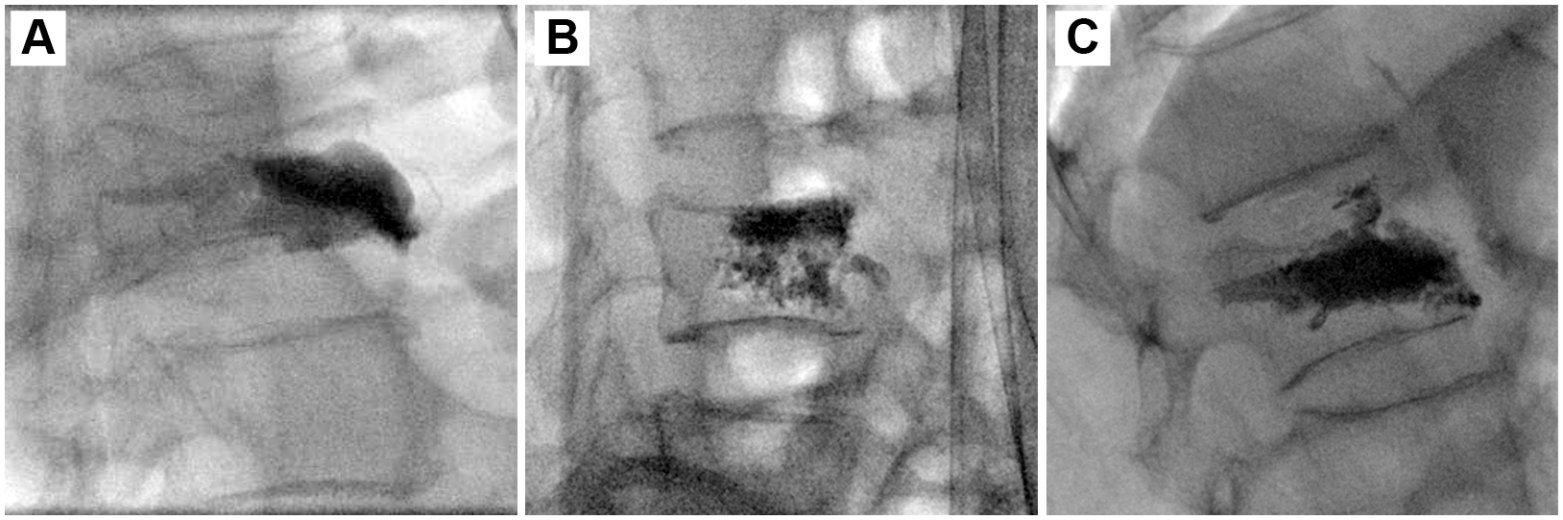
Radiographs of PMMA cement leakage. (A) In a case of PVP treatment, PMMA leaked into vein in a linear way. (B) In a case of PVP treatment, PMMA leaked outside of the treated vertebra. (C) In a case of PKP treatment, PMMA leaked from the broken endplate to the intervertebral disc.
